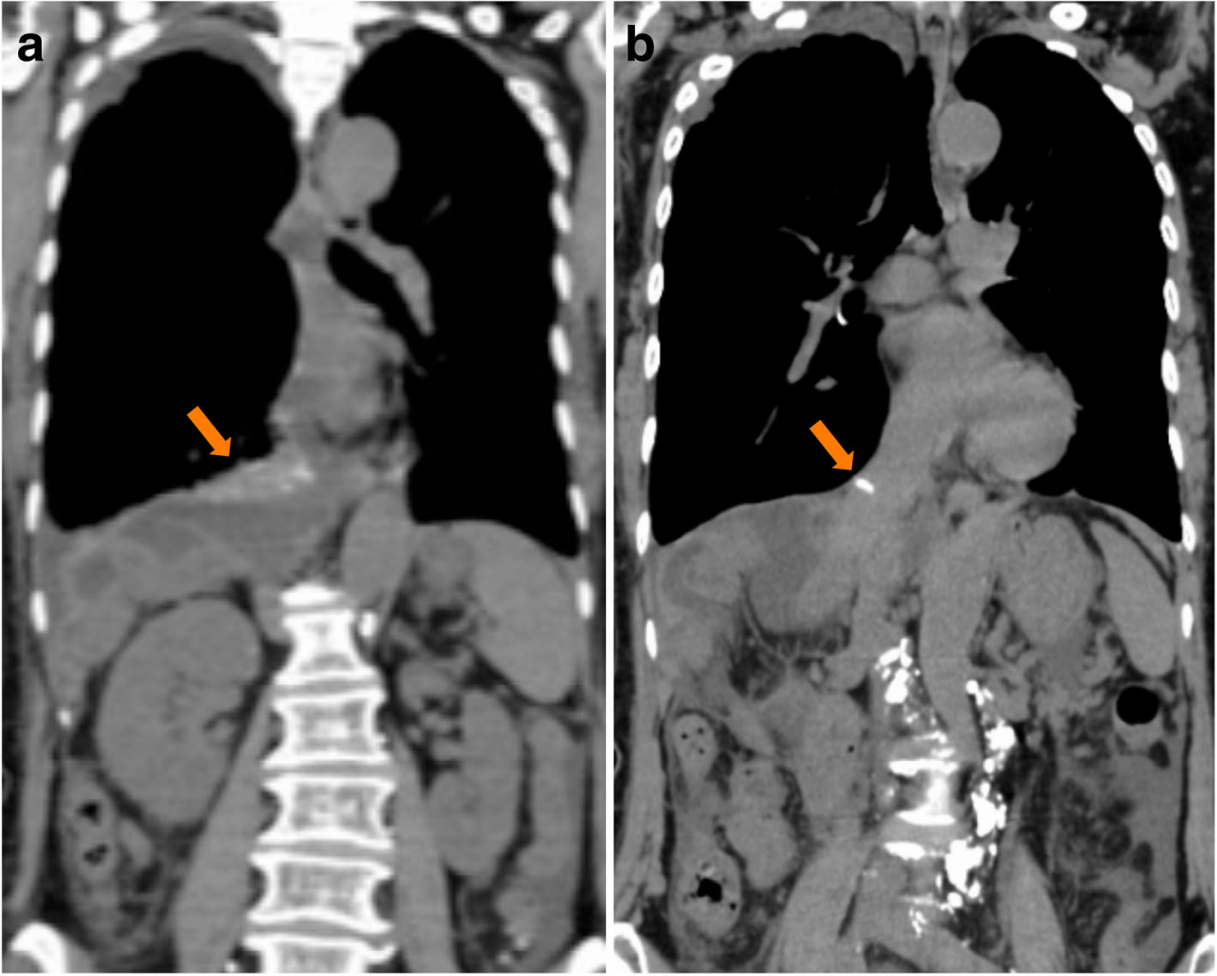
a Post-procedure computed tomography revealing extravasation of Lipiodol (ethiodized oil) adjacent to the right mediodorsal pleural space on the diaphragm, but the location of the leak was not identified. The arrow pointing to the extravasation of Lipiodol (ethiodized oil). b Computed tomography revealing accumulation of Lipiodol (ethiodized oil) near the staple line of the stump of the right hepatic vein. The arrow pointing to the staple line of the stump of the right hepatic vein
